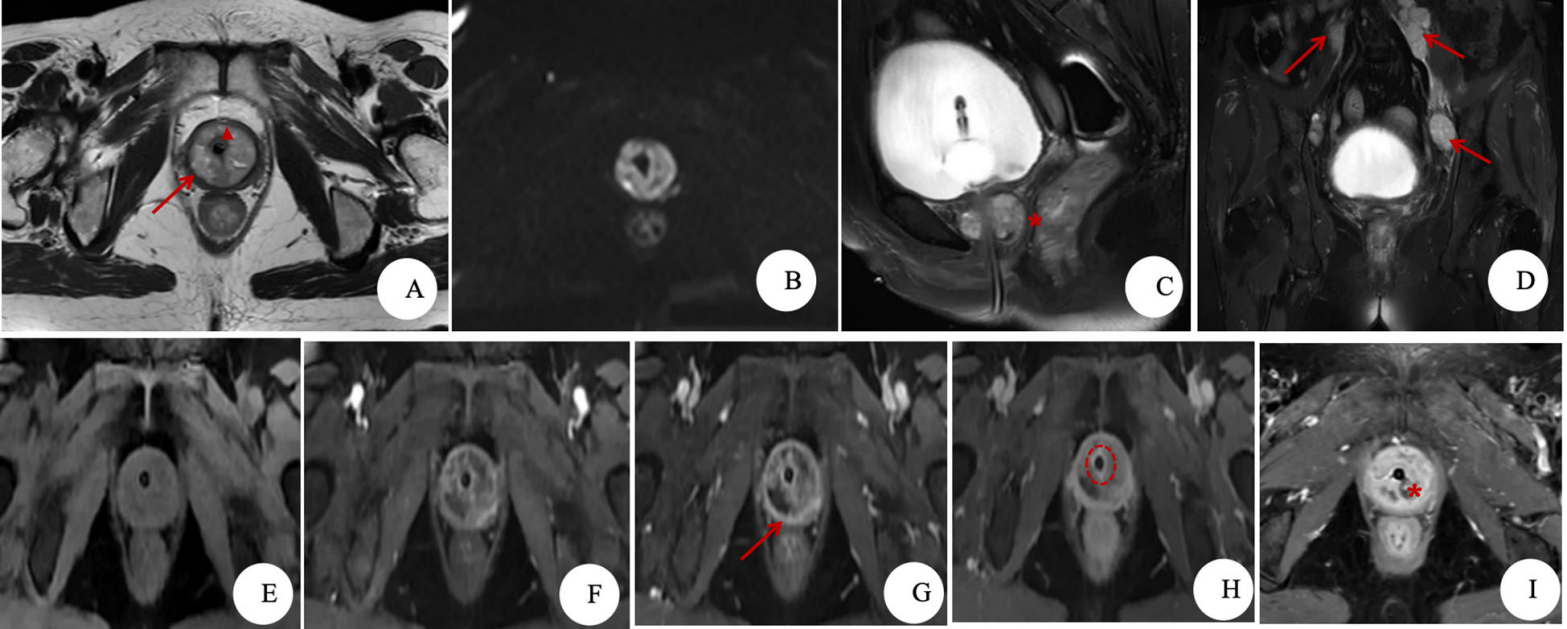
MRI findings of clear cell adenocarcinoma of the urethra (CCAU). (A) Axial T2WI: An annular, round-like mass is visible around the upper urethra, demonstrating slightly high signal intensity (compared to muscle). Within the mass, small patchy areas of high signal and punctate low signal foci (red triangle) are observed, surrounded by a low-signal capsule (red arrow) with well-defined margins. (B) DWI, *b* = 1,000 s/mm²: The mass shows restricted diffusion, with an ADC value of 0.79 × 10^-^³ mm²/s. (C) Sagittal T2WI: The mass exhibits clear boundaries with the bladder and vagina (red star), with posterior displacement of the vaginal wall. (D) Coronal T2WI: Multiple enlarged lymph nodes are visible along the bilateral iliac vessels (red arrow). (E) Pre-contrast mask: The mass shows iso- to slightly low signal intensity (compared to muscle). (F–I) DCE series: The tumor is hypervascular and demonstrates progressive heterogeneous enhancement. Non-enhanced necrotic areas are noted within the mass (red triangle), with prominent capsular enhancement (red arrow). Circumferential enhancement of the urethral wall is visible on certain sequences (red dashed circle), and the local urethral morphology appears irregular (red star).
